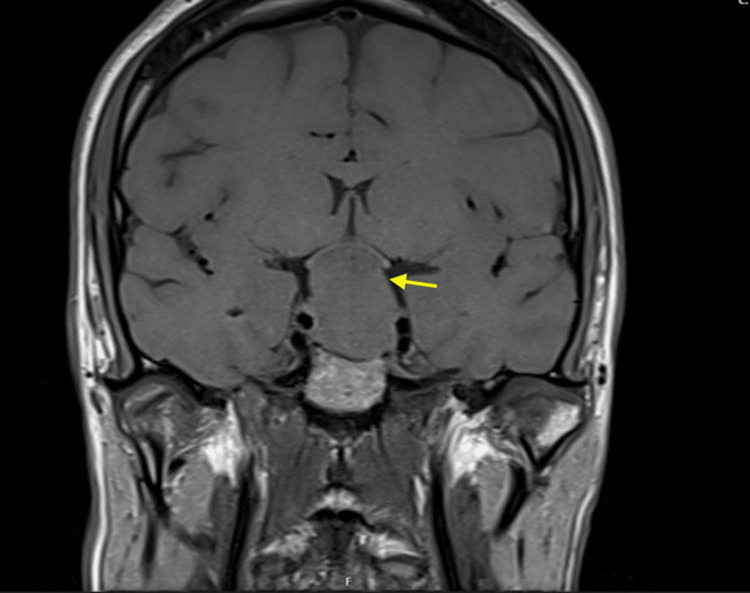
Coronal view of the preoperative pituitary MRI Arrow pointing towards a 5cm giant pituitary adenoma compressing the optic chiasm.
